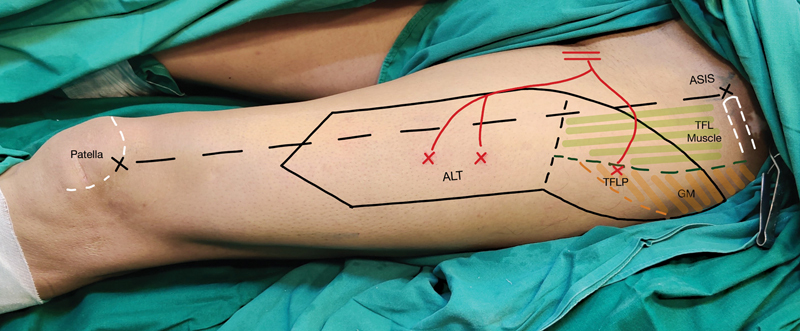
Surface marking of the ALT and TFL flap territory and perforator location. A noncommittal incision which is 1.5 cm medial to the vascular axis of the ALT flap (anterior superior iliac spine and the superolateral patella). To approach the TFL perforator, the same incision is extended posterosuperiorly guided by the handheld Doppler signal. ALT, anterolateral thigh; TFL, tensor fascia lata; TFLP, tensor fascia lata perforator.
